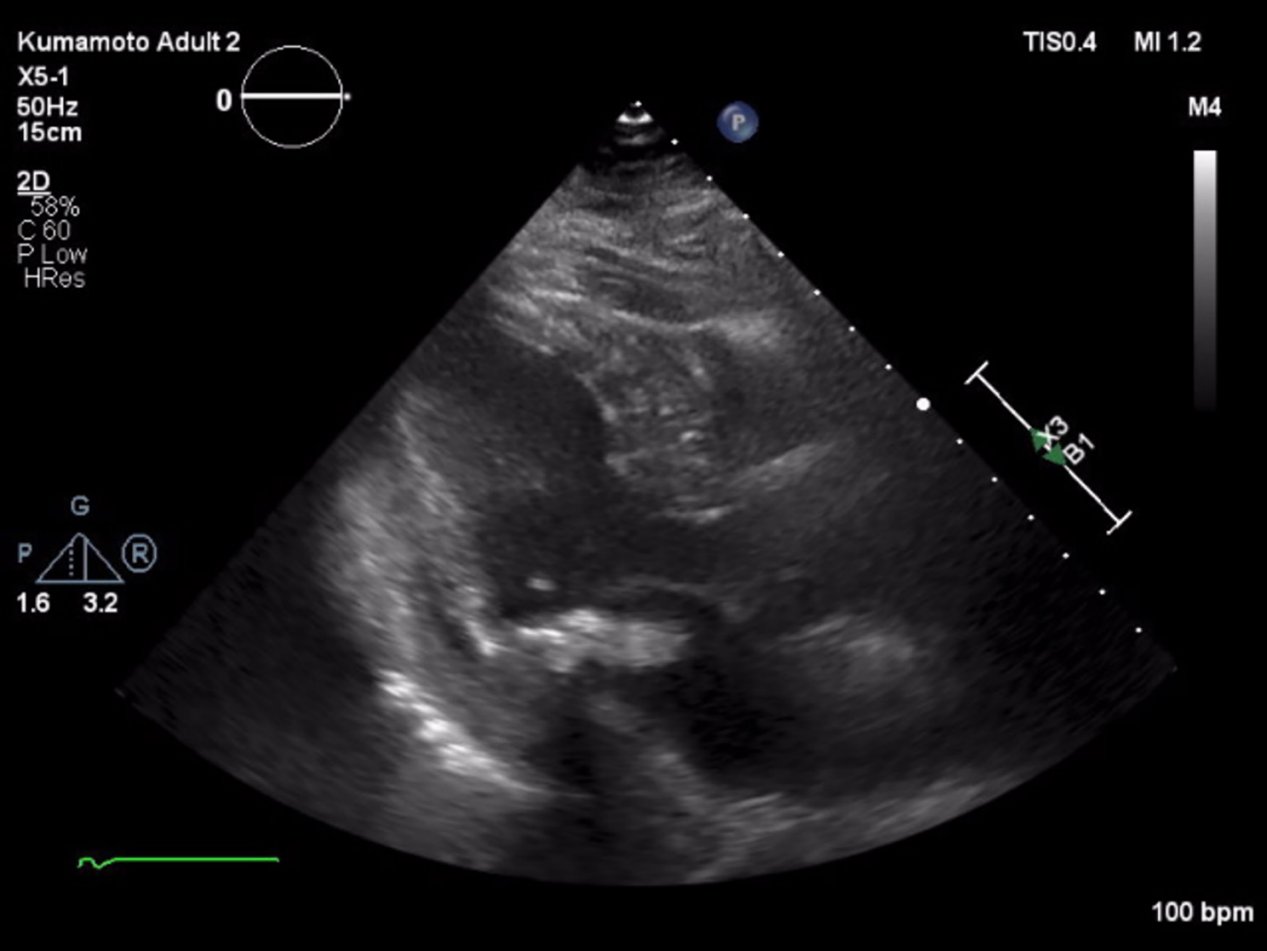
Two‐dimensional transthoracic echocardiography scan shows an inhomogeneous mass attached to posterior annuls with calcification.
